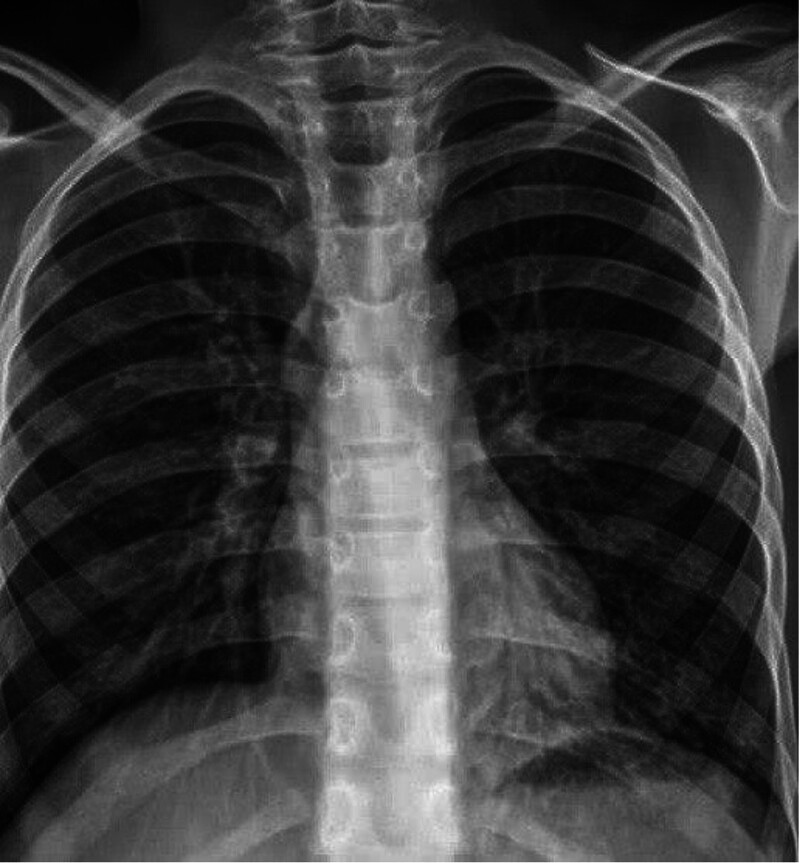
Simple chest X-ray showing no abnormalities.
